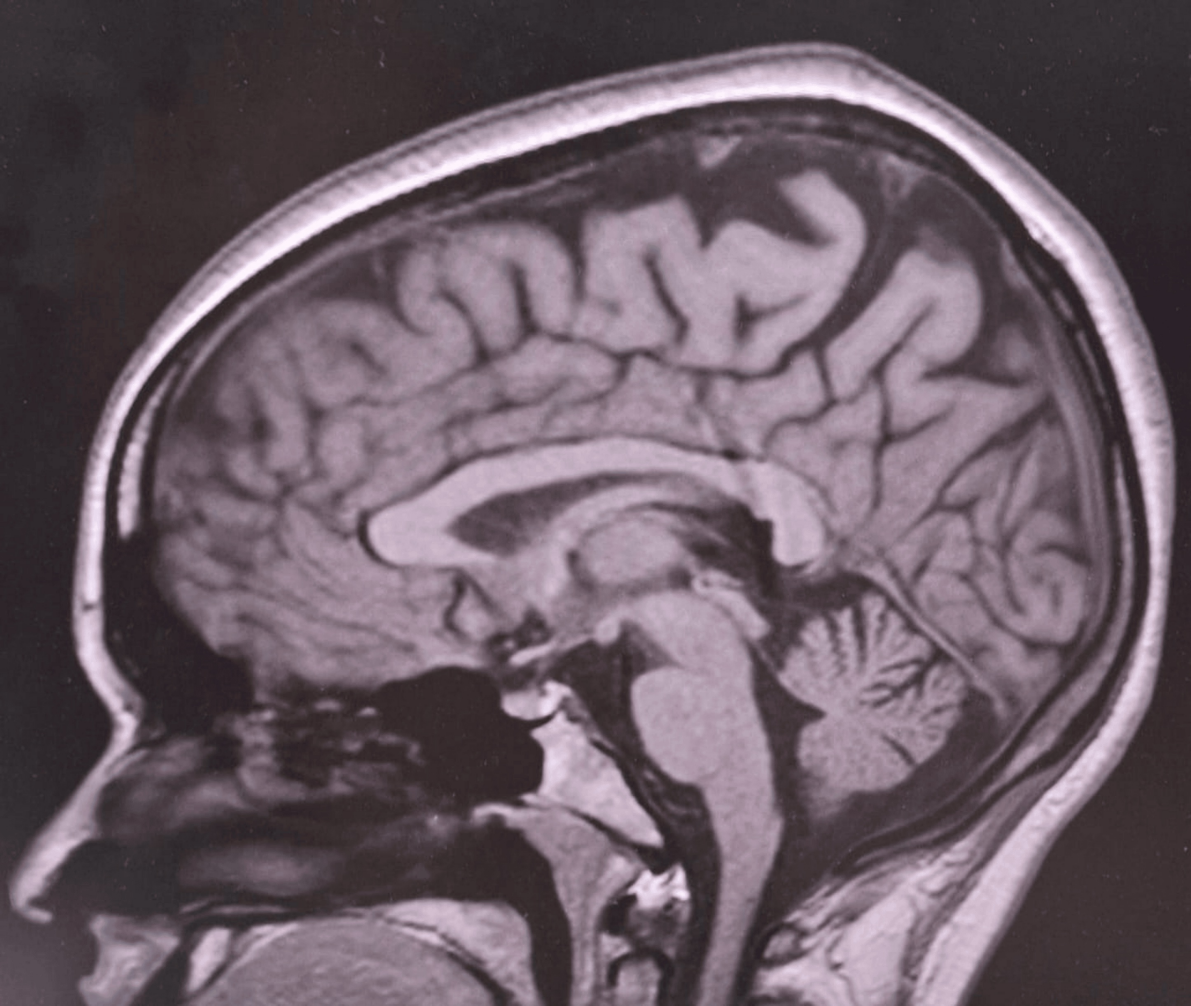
MRI of the patient's brain The brain MRI did not show any well-defined round lesion, which is characteristic of neurocysticercosis.
